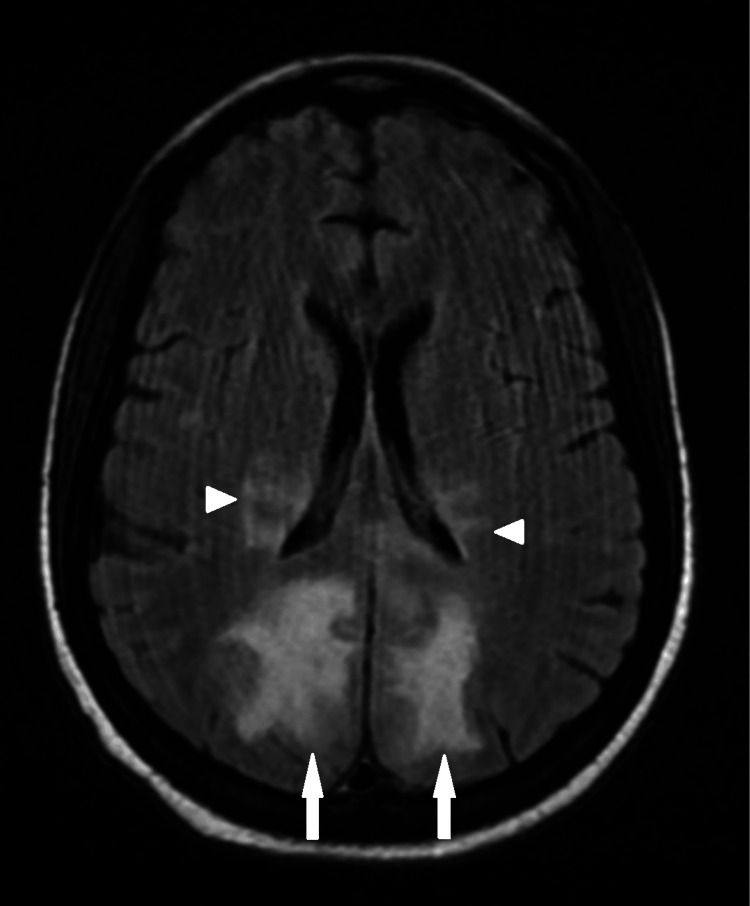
Axial FLAIR demonstrates white matter hyperintensities in the posterior parietal (arrowhead) and occipital lobes (arrow) that spare the gray matter.
